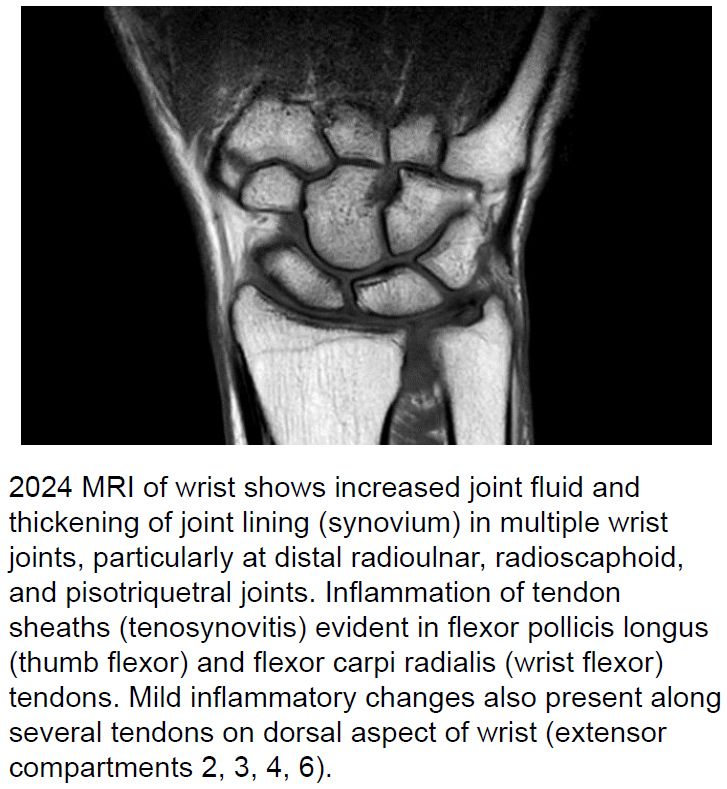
2024 MRI Image of Ross River Virus-Infected Australian Service Member's Right Wrist, Indicating Capsulosynovitis
